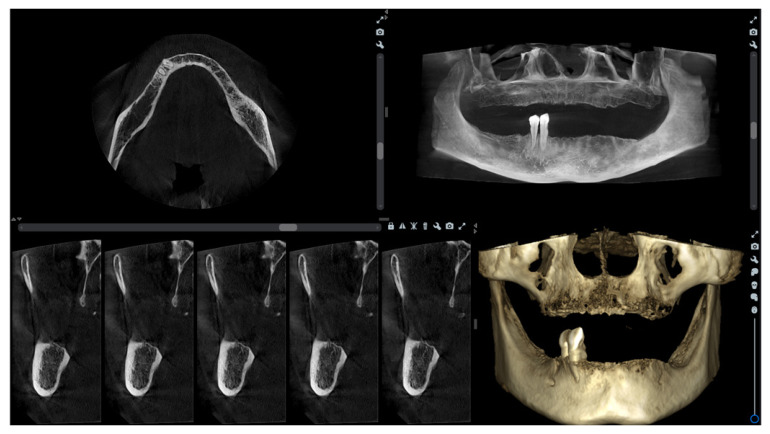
Initial CBCT evaluation of a fully edentulous patient used for treatment planning. Bone volume, anatomical limitations, and prosthetic space were assessed prior to digital implant planning.
